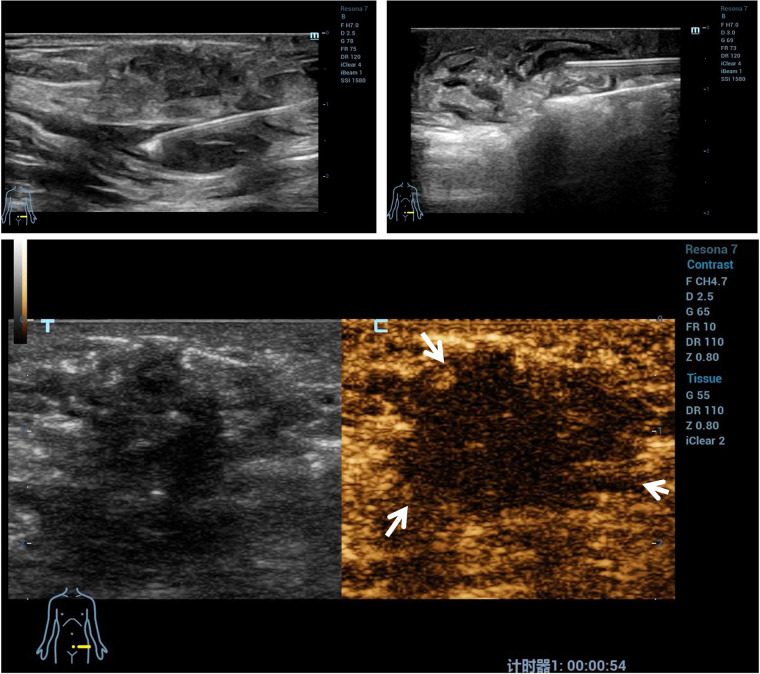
A representative case of microwave ablation treatment. Microwave ablation was performed, and a gasification reaction of the hyperechoic cloud was observed inside the lesion region. The contrast-enhanced ultrasound imaging of AWE nodules shows no contrast agent filling in the nodules. AWE, abdominal wall endometriosis.
